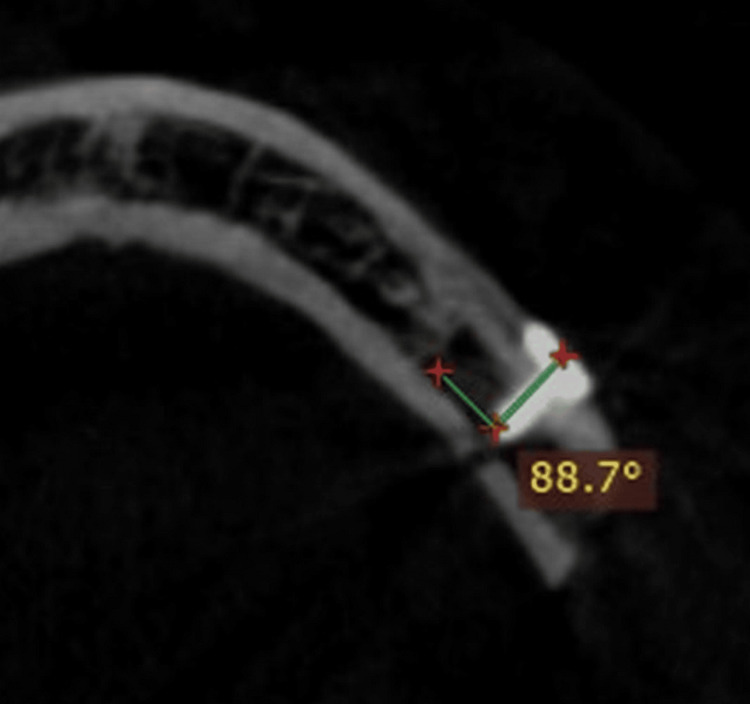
CBCT image showing angulation of screw fixation with a conventional screwdriver CBCT, cone beam computed tomography
